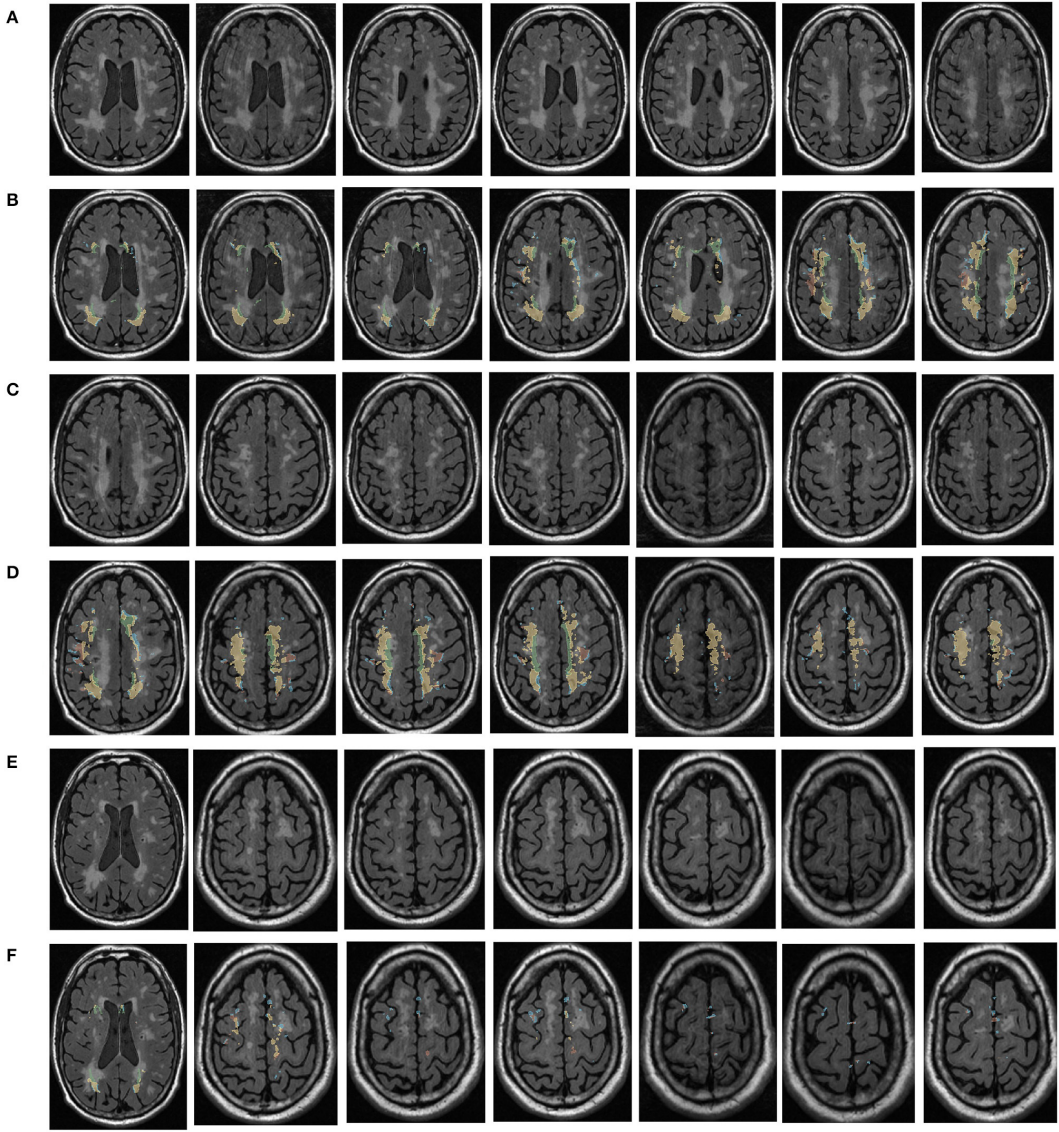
Visualization of white matter hyperintensity coverage. Rows (A, C, E) represent MRI visualizations of the brain not covered by cerebral white matter hyperintensity, rows (B, D, F) represent MRI images of cerebral white matter hyperintensity in brain regions that have produced a certain regional volume.
